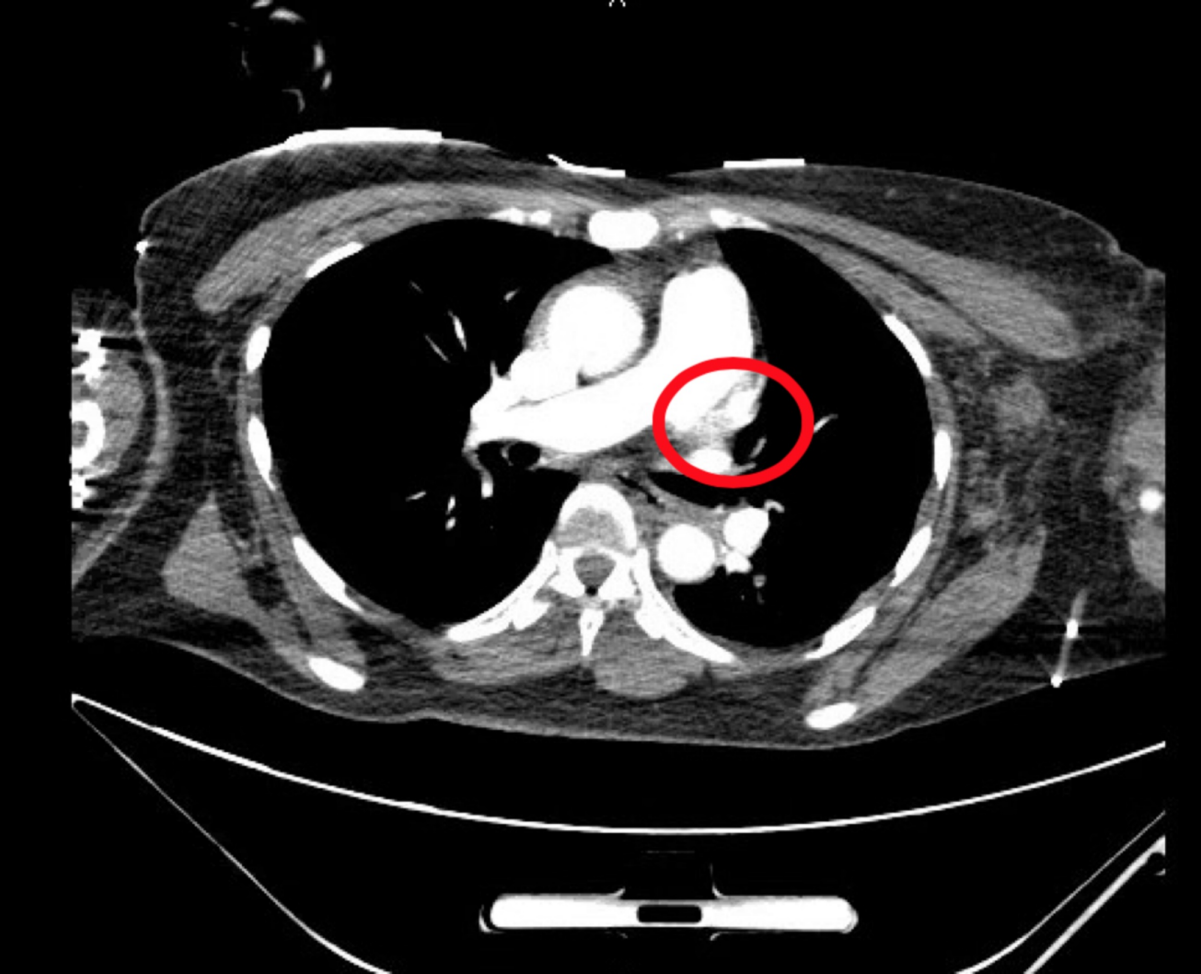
Computed tomography angiogram shows a large filling defect in the main left pulmonary artery consistent with a massive pulmonary embolism. Note also dilation of the pulmonary trunk and right main pulmonary artery.
